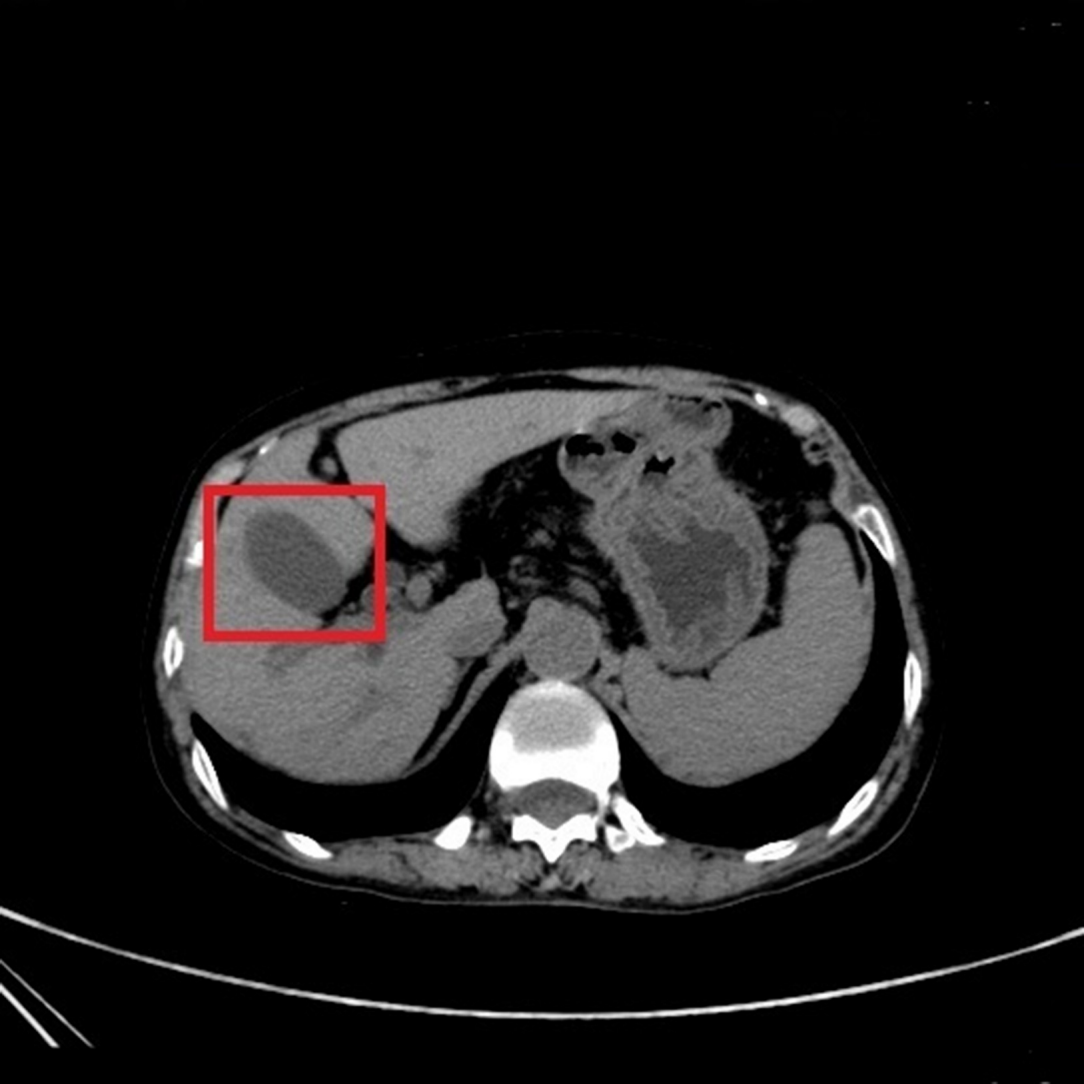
The CT image of a healthy person.
